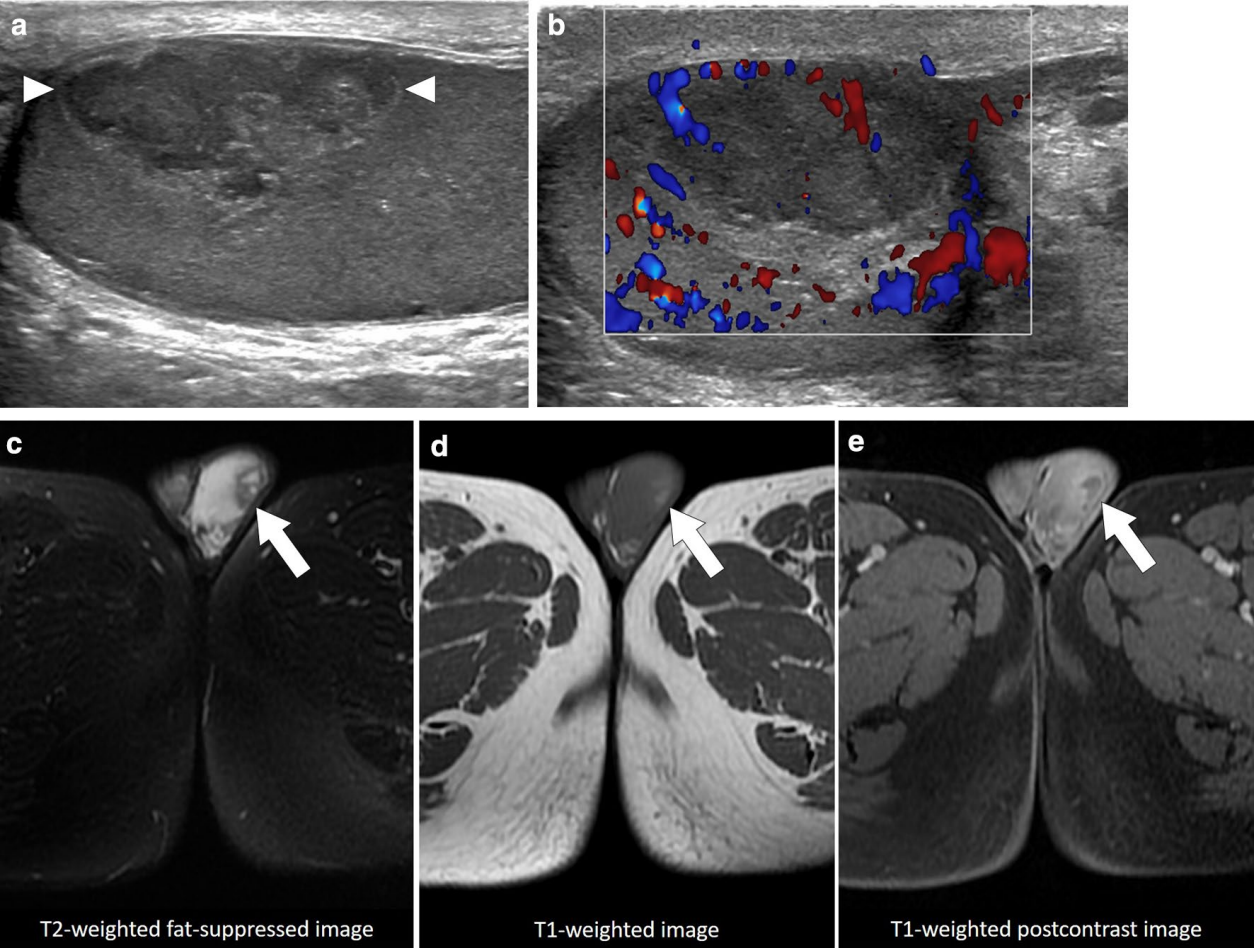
Testicular hematoma. A 30-year-old male presenting after a fall from height for which he did not seek any medical care. Ten days after the initial event, he presented with a palpable left testicular mass. a Gray scale US shows a heterogeneous hypoechoic lesion in the left testis (arrowheads). b There was mild internal vascularity within this lesion on color Doppler US exam. Hematoma was considered as the initial diagnosis but as testicular tumor could not be ruled out an MRI order was placed. c-d Axial plane T2W fat-suppressed and T1W images show high T1 and T2 signal intensities within the central part of the lesion (arrows) which was thought to represent hemorrhage in subacute phase. The peripheral low T2 signal intensity was considered hemosiderin deposition. e Axial plane postcontrast MR image shows no internal enhancement within the central part (arrow). During the follow-up, the lesion completely regressed, leaving mild testicular atrophy (not shown)
